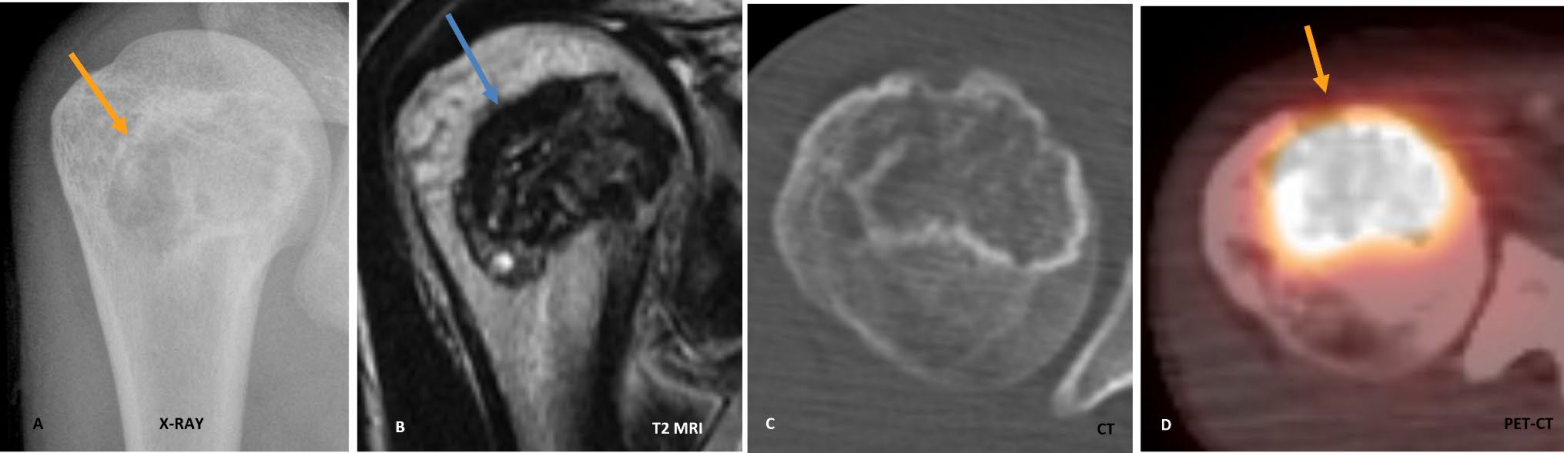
Typical imaging features of chondroblastoma on radiograph, MRI, CT, and PET–CT. A Radiograph demonstrates an osteolytic epi-metaphyseal lesion with narrow zone of transition, sclerotic rim, and internal calcification. B T2W coronal image through the humeral head shows a T2 hypointense lesion with occasional T2 hyperintense foci. C Axial CT image in bone window demonstrates sclerotic rim and internal chondroid type calcification. D Fused transverse PET–CT image shows intense FDG-avidity within the lesion
